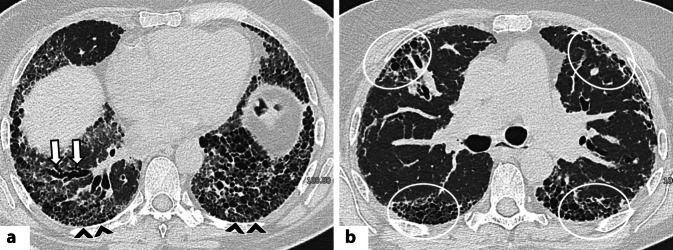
a Axiale hochauflösende Computertomographie (HRCT) einer Patientin mit systemischer Sklerose (SSc): Übergang von einem NSIP-Muster (nichtspezifische interstitielle Pneumonie) – erkennbar an zentralen Bronchiektasen im rechten Unterlappen (*weiße Pfeile*) umgeben von Milchglasverdichtungen – hin zu einem UIP-Muster (gewöhnliche interstitielle Pneumonie) mit bilateralem, subpleuralem Honeycombing (*schwarze Pfeilköpfe*). b Axiale HRCT-Aufnahme bei einer Patientin mit SSc mit dem sog. „four-corner sign“: Zeichen einer pulmonalen Fibrose (einschließlich Honeycombing) in den vier „Ecken“ der Lunge – anterolaterale Areale der Oberlappen und posterosuperioren Arealen der Unterlappen (*weiße Kreise*)
